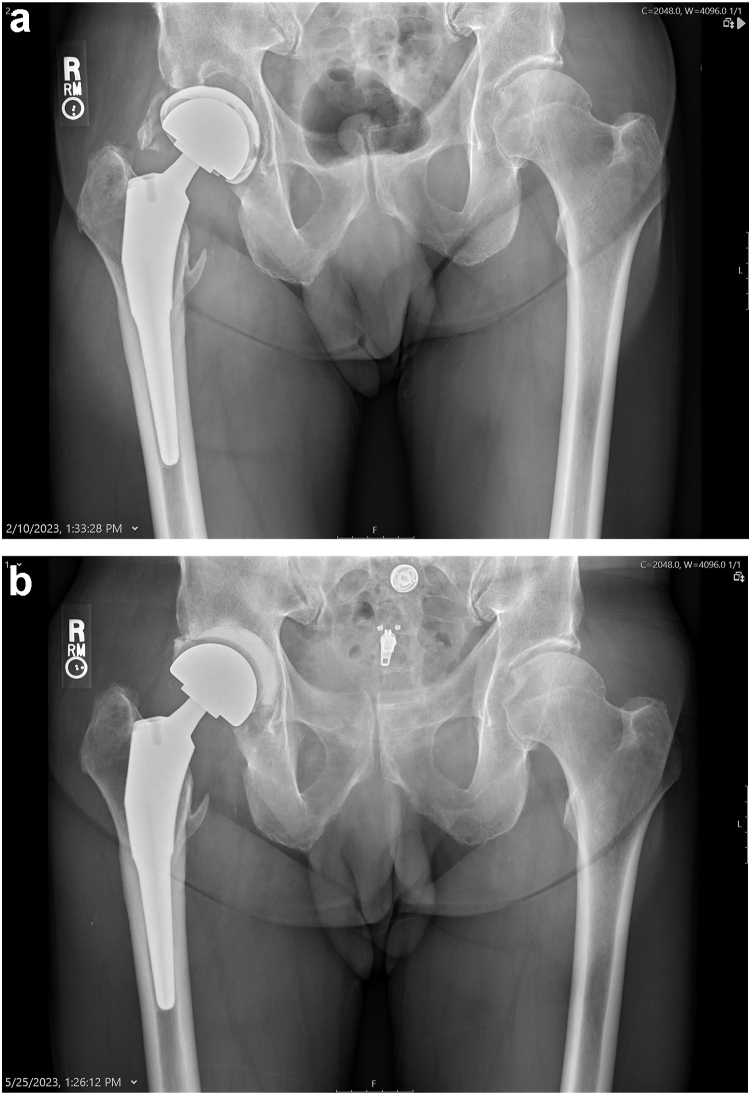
(a) Photograph of a chronic S. lugdunensis infection of a total hip arthroplasty in a 76-year-old man. (b) The postoperative anteroposterior pelvis radiograph shows the acetabular shell has been removed and replaced with an antibiotic-containing PUR liner supported by antibiotic cement.
